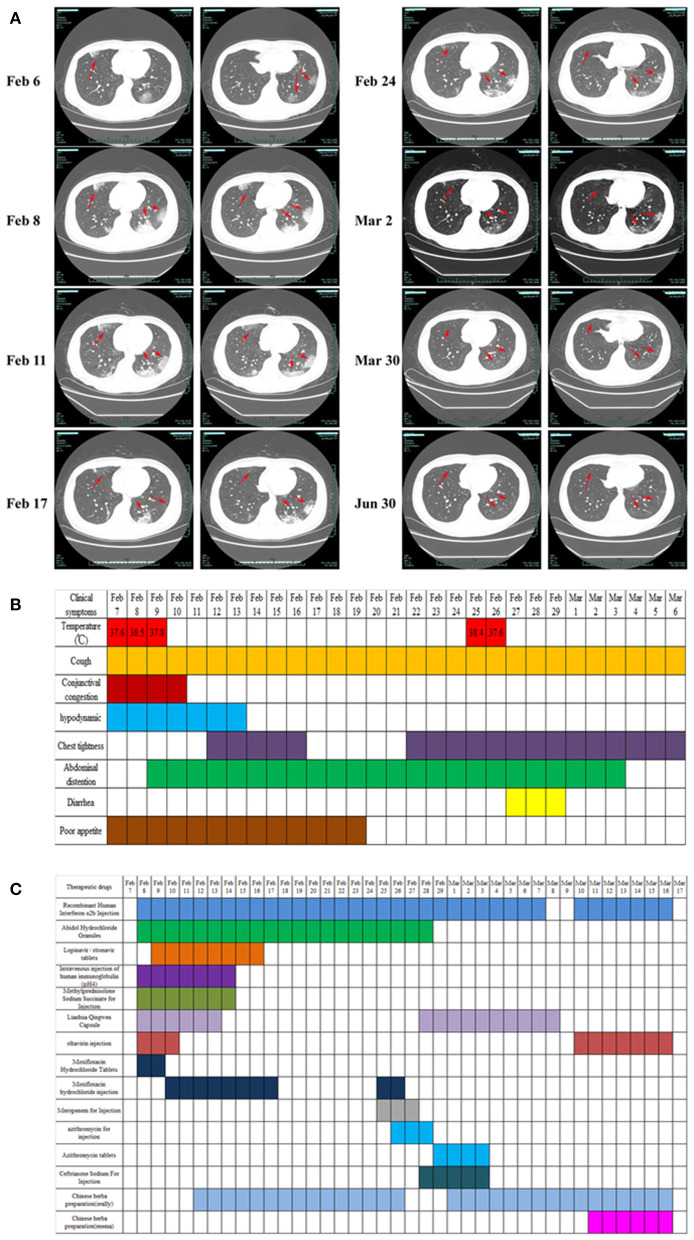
Clinical imaging, symptoms, and drug treatment data of patients with COVID-19. (A) CT image changes of patients with COVID-19 from first visit to discharge. (B) Changes in clinical symptoms in patients with COVID-19 during treatment after admission. (C) Medication information for patients with COVID-19 during hospitalization. Drug dosage: Recombinant human interferon α2b injection (6 million U, twice daily, aerosol inhalation); abidol hydrochloride granule (0.2 g, 3 times daily, oral administration); lopinavir/ritonavir (2 capsules, twice daily, oral administration); intravenous injection of human immunoglobulin (pH 4) (20 g, once daily, intravenous injection); methylprednisolone sodium succinate for injection (40 mg, once daily, subcutaneous injection); Lianhua Qingwen capsule (1.4 g, 3 times daily, oral administration); ribavirin injection (0.5 g, twice daily, intravenous injection); moxifloxacin hydrochloride tablets (400 mg, once daily, oral administration); moxifloxacin hydrochloride injection (0.4 g, once daily, intravenous injection); meropenem for injection (1 g, once per 8 h, intravenous injection); azithromycin for injection (0.5 g, once daily, intravenous injection); azithromycin tablets (0.5 g, once daily, oral administration); ceftriaxone sodium for injection (2 g, once daily, intravenous injection).
